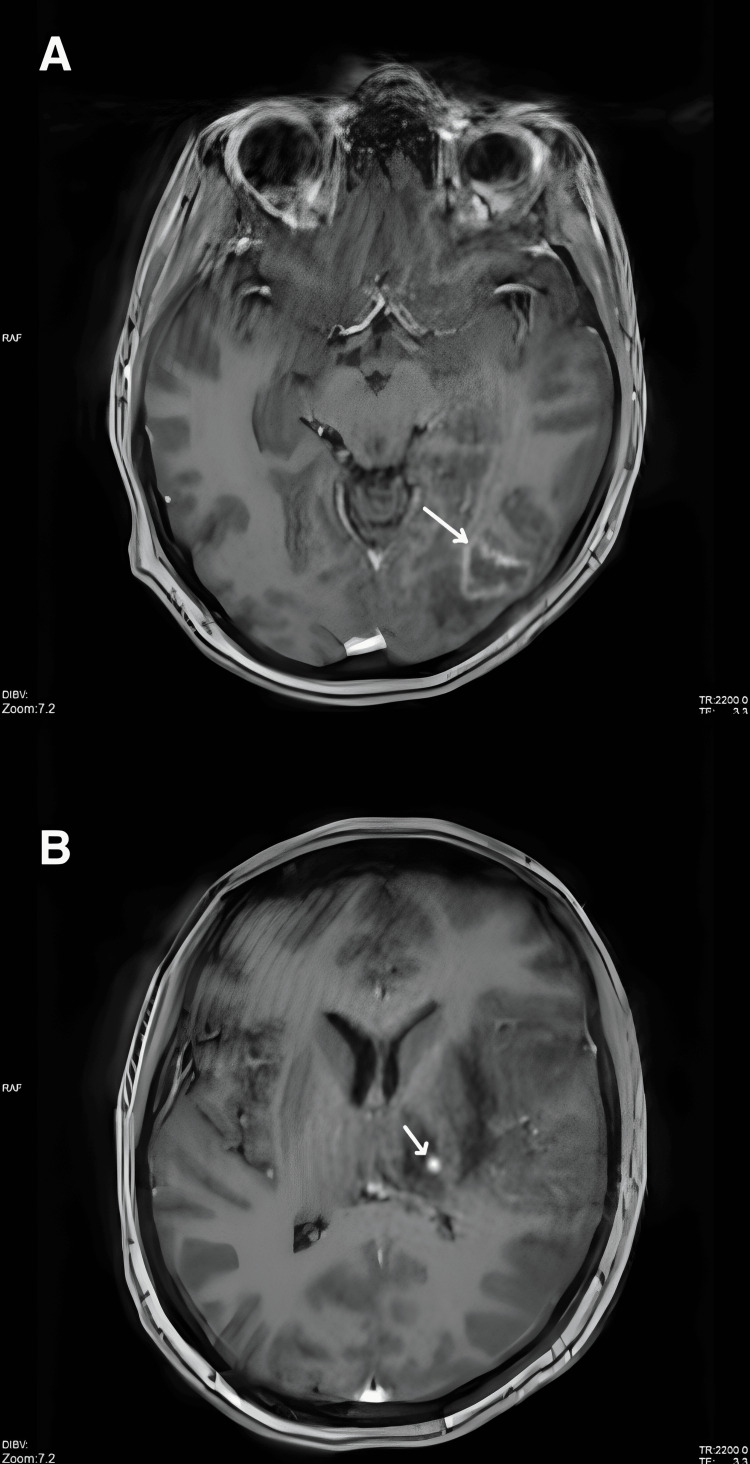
Repeat MRI of the brain (A) Progression of the contrast-enhancing lesion in the left occipital lobe (white arrow). (B) Newly developed contrast-enhancing nodule in the left thalamus (white arrow), suggestive of disease progression.
